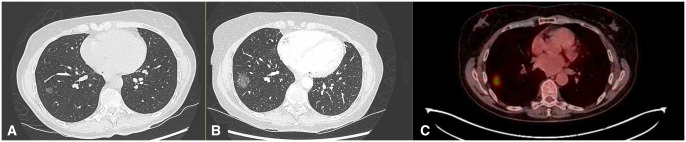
(A) Baseline CT-Scan (B) Preoperative CT-Scan 2 (C) Preoperative TEP-Scan.
